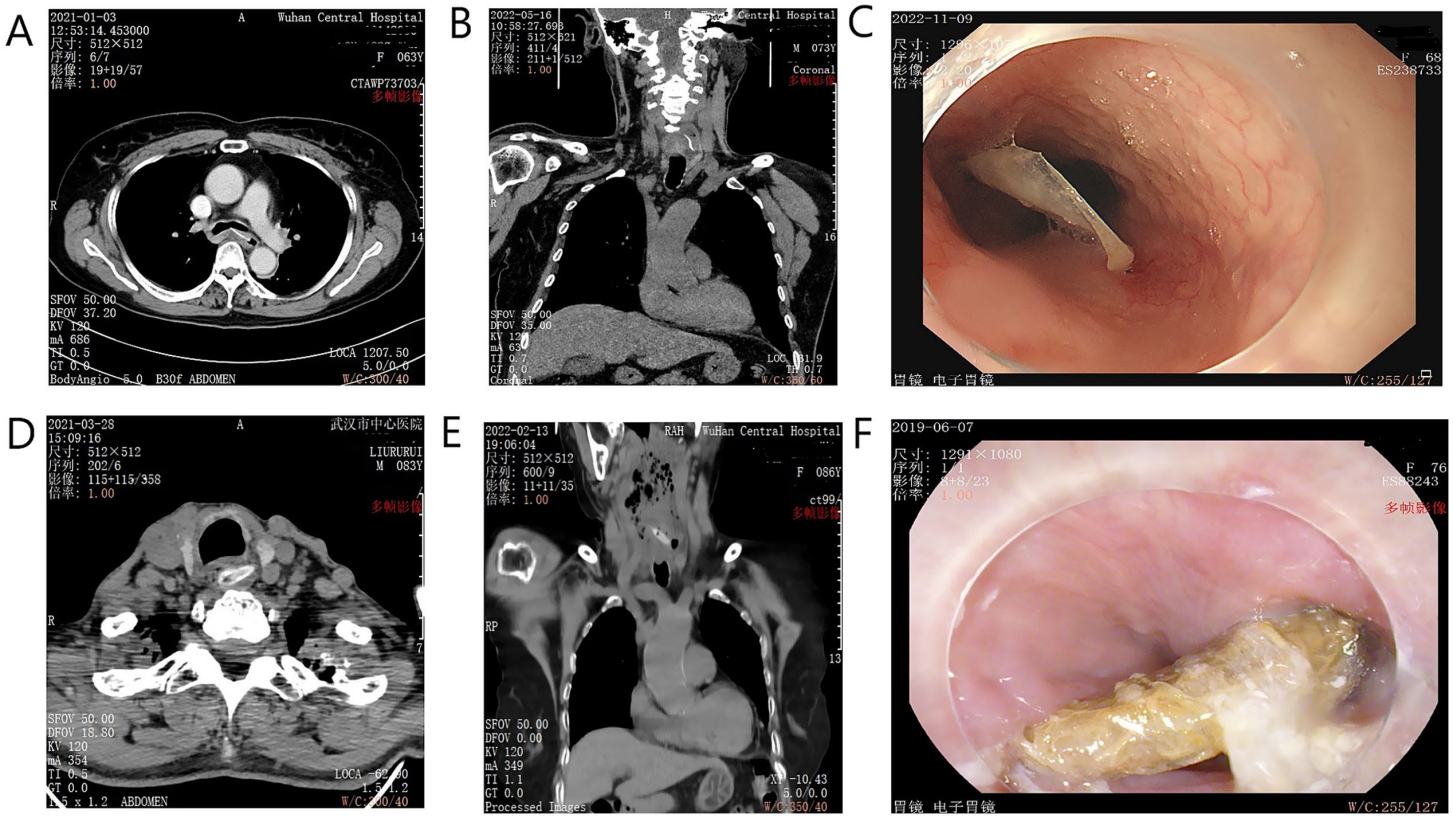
Examples of sharp foreign bodies identified by CT and endoscopy. (A) Axial CT scan showing a fish bone penetrating the esophageal wall. (B) Coronal CT view of a similar injury. (C) Endoscopic view of an impacted fish bone. (D) Axial CT showing a jujube pit in the cervical esophagus. (E) Coronal CT view of an impacted jujube pit causing peri-esophageal inflammation. (F) Endoscopic view of a large, impacted jujube pit.
